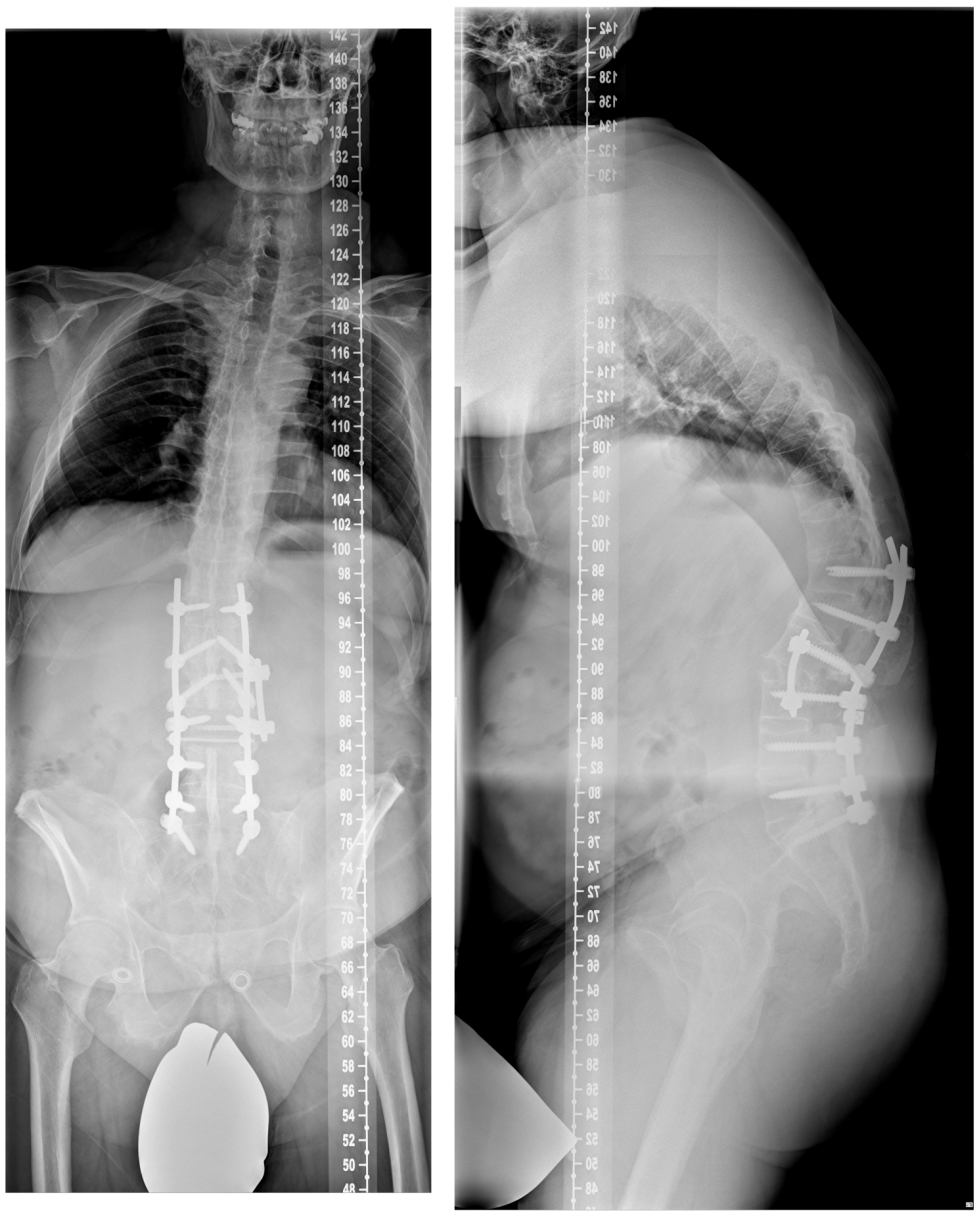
The whole spine conventional X-rays after the second surgery reveal an improved sagittal profile, however, the initially planned and desired amount of correction has not been reached completely.
